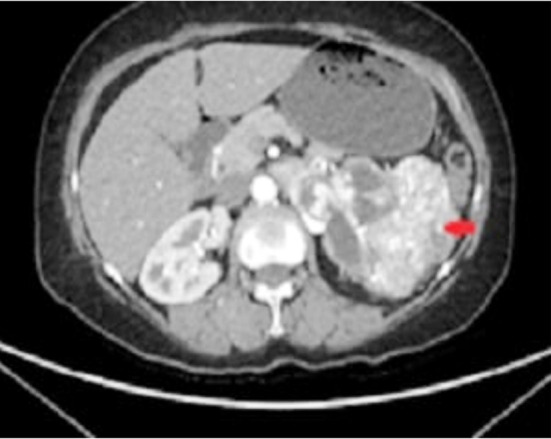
CT chest abdomen pelvis with contrast (Red arrows shows vascular space occupying lesion almost replacing the entire left kidney and invading the pelvicalyceal system, reaching the left proximal ureter.).
